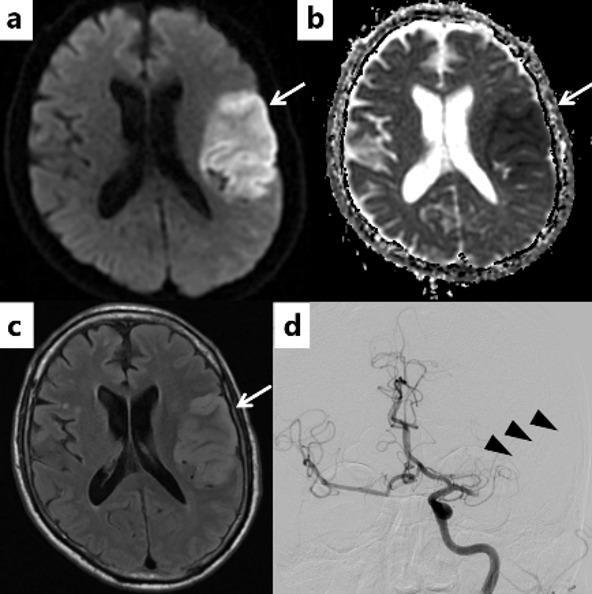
Stroke. a DWI, b ADC map, c FLAIR, d DSA.A man in his 50s with type 2 DM had sudden-onset right-sided hemiplegia. MRI (a–c) reveals a large left MCA territory infarct (arrow). DSA reveals left MCA obstruction (arrowhead). Intra-arterial thrombolysis resulted in complete left MCA recanalization. Abbreviations: DM, diabetes mellitus; FLAIR, fluid-attenuated inversion recovery; MCA, middle cerebral artery; DSA, digital subtraction angiography; MRI, magnetic resonance imaging; DWI, diffusion-weighted imaging
